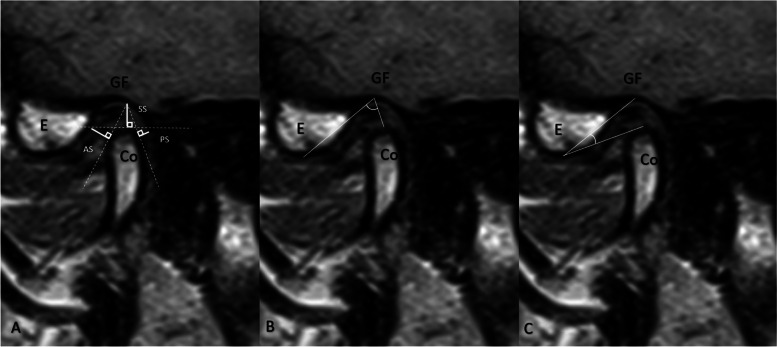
TMJ measurements in MRI. A Distance measurements. B Antero-posterior Angle (APA) C. Supero-inferior Angle (SIA). MRI: Magnetic resonance imaging, Co: Condyle, E: Articular eminence, GF: Glenoid Fossa, AS: Anterior Space, SS: Superior Space, PS: Posterior Space
